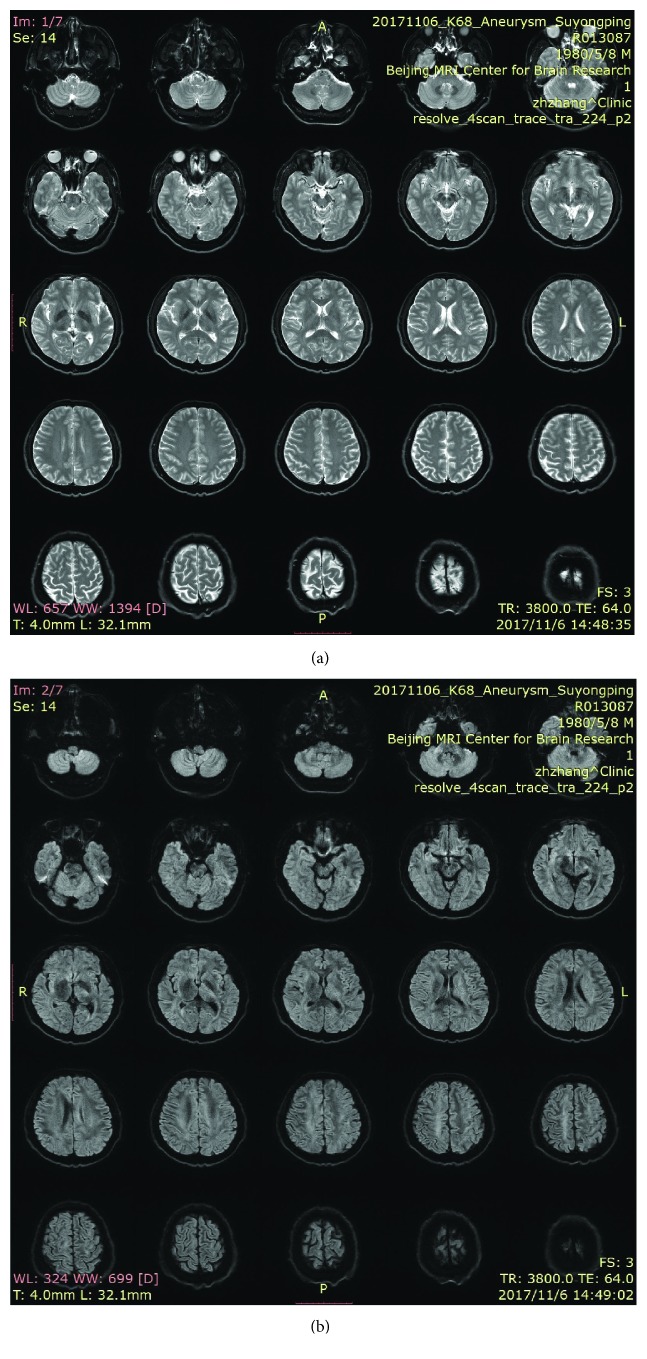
Postoperative head nuclear magnetic resonance imaging.
